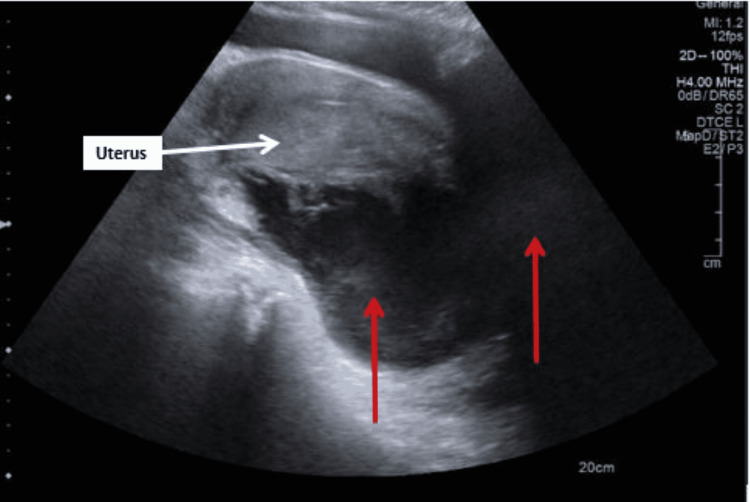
Transvaginal ultrasound image 2 showing 12cm by 6cm and 6cm by 4cm hypoechogenic lesions (indicated by red arrows)
